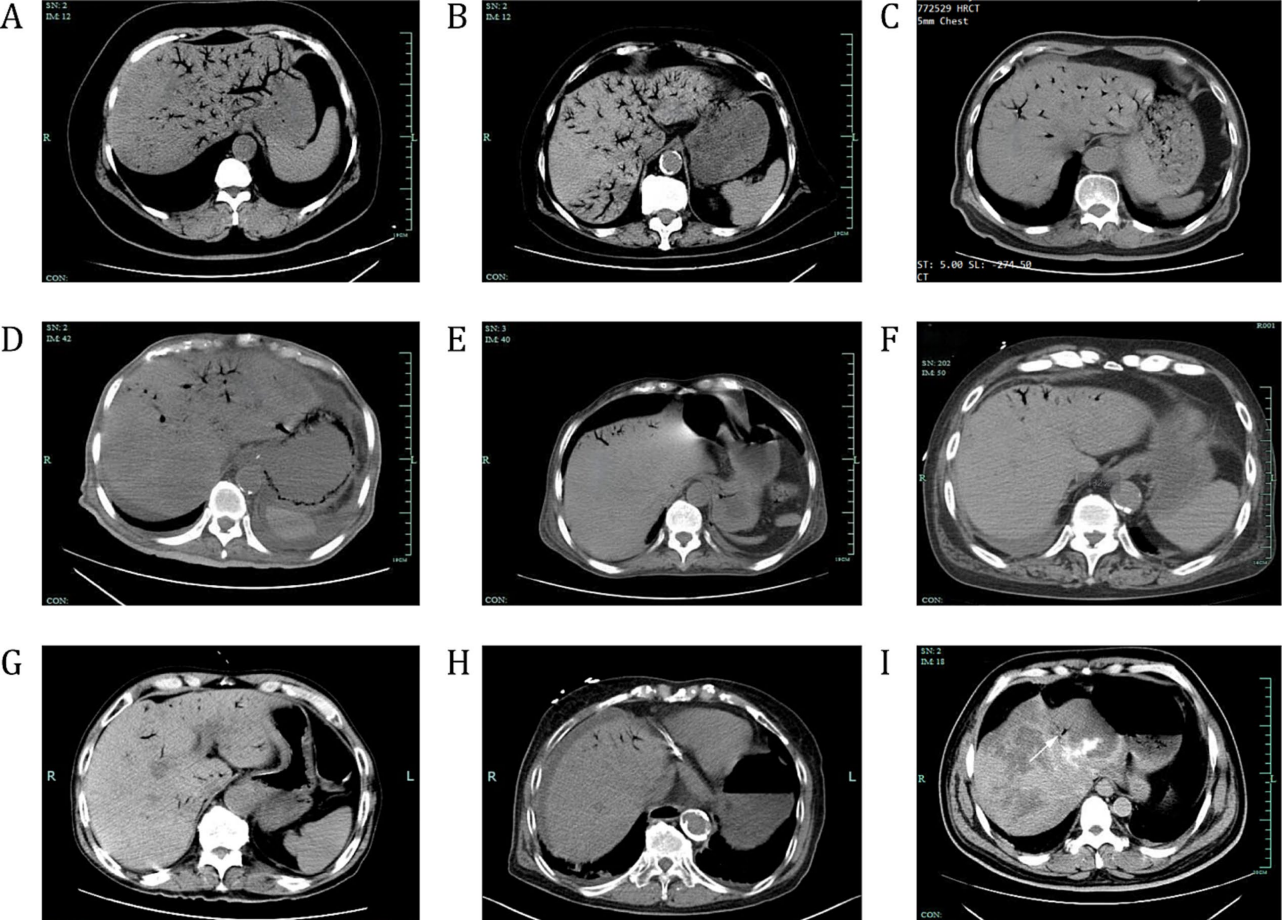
Nine patients with HPVG. CT scan of nine patients with varying degrees of HPVG. (A,B) Abdominal CT scans show extensive gas throughout the entire portal venous system. ‘Entire’ indicates that gas is present in two or more hepatic segments, with the gas occupying a relatively large area within these segments. (C–G) Gas is present in more than two segments of the liver. ‘Two segments’ means that gas is present in two or more hepatic segments, but the area occupied by the gas within each of these segments is relatively small. (H,I) Minimal gas is found within a 2 cm range of the liver capsule or within a single liver segment. HPVG, hepatic portal venous gas; CT, computed tomography.
